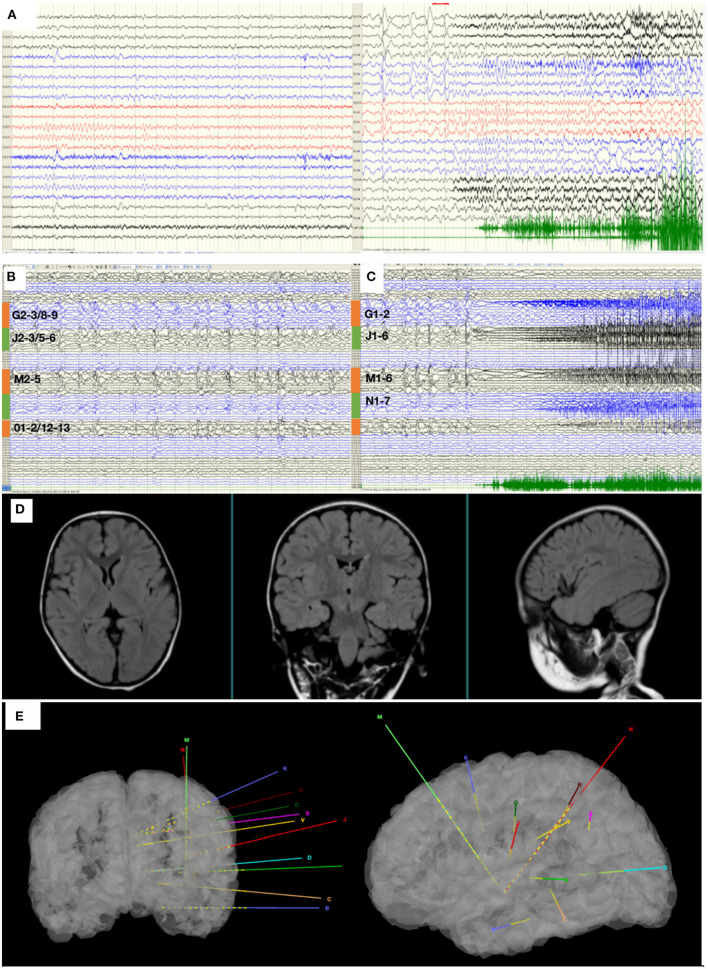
(A) The interictal and ictal scalp VEEG. Scalp VEEG showed seizure onset with stiffening of right limb, apnea, salivation, waving of left limb. (B) SEEG showed all the inter-ictal discharges were located in insular-opercular cortex (J2-3/5-6, M2-5, G2-3/8-9, 01-2/12-13). (C) SEEG showed that the seizures onset zones originated from the insular-opercular cortex (J1-6, G1-2, M1-6, N1-7) with spike-wave in fast activities. The Semiology is stiffening of right limb, apnea, salivation, waving of left limb. (D) MRI images of the patient before the first intracranial electrode implantation. (E) The position of twelve electrodes.
